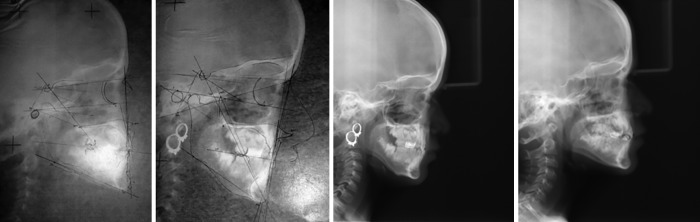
(A) Pretreatment cephalometric analysis; (B) posttreatment cephalometric analysis; (C) pretreatment radiograph; and (D) posttreatment radiograph
